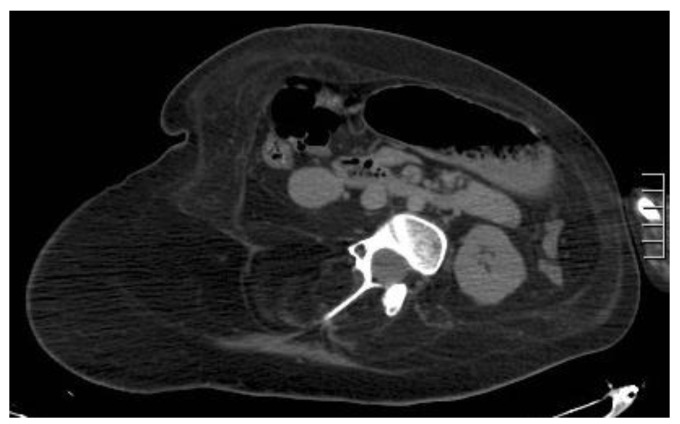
A 23-year-old patient with Duchenne’s syndrome. Axial, not-enhanced CT scan demonstrates complete fat replacement of all abdominal wall muscles with obligated decubitus, as commonly seen in cases of Duchenne’s syndrome patients reaching the ages of 20–30.
